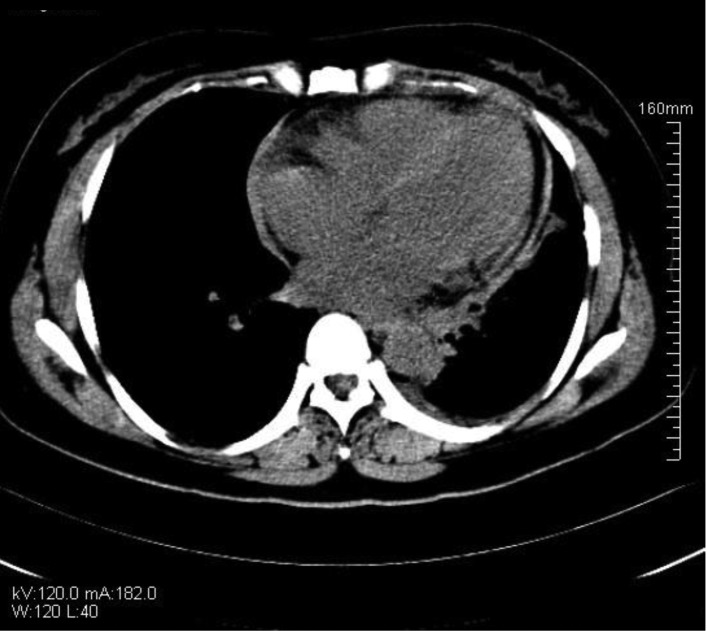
CT revealing a small amount of pericardial effusion and disappearance of the pleural effusion.
